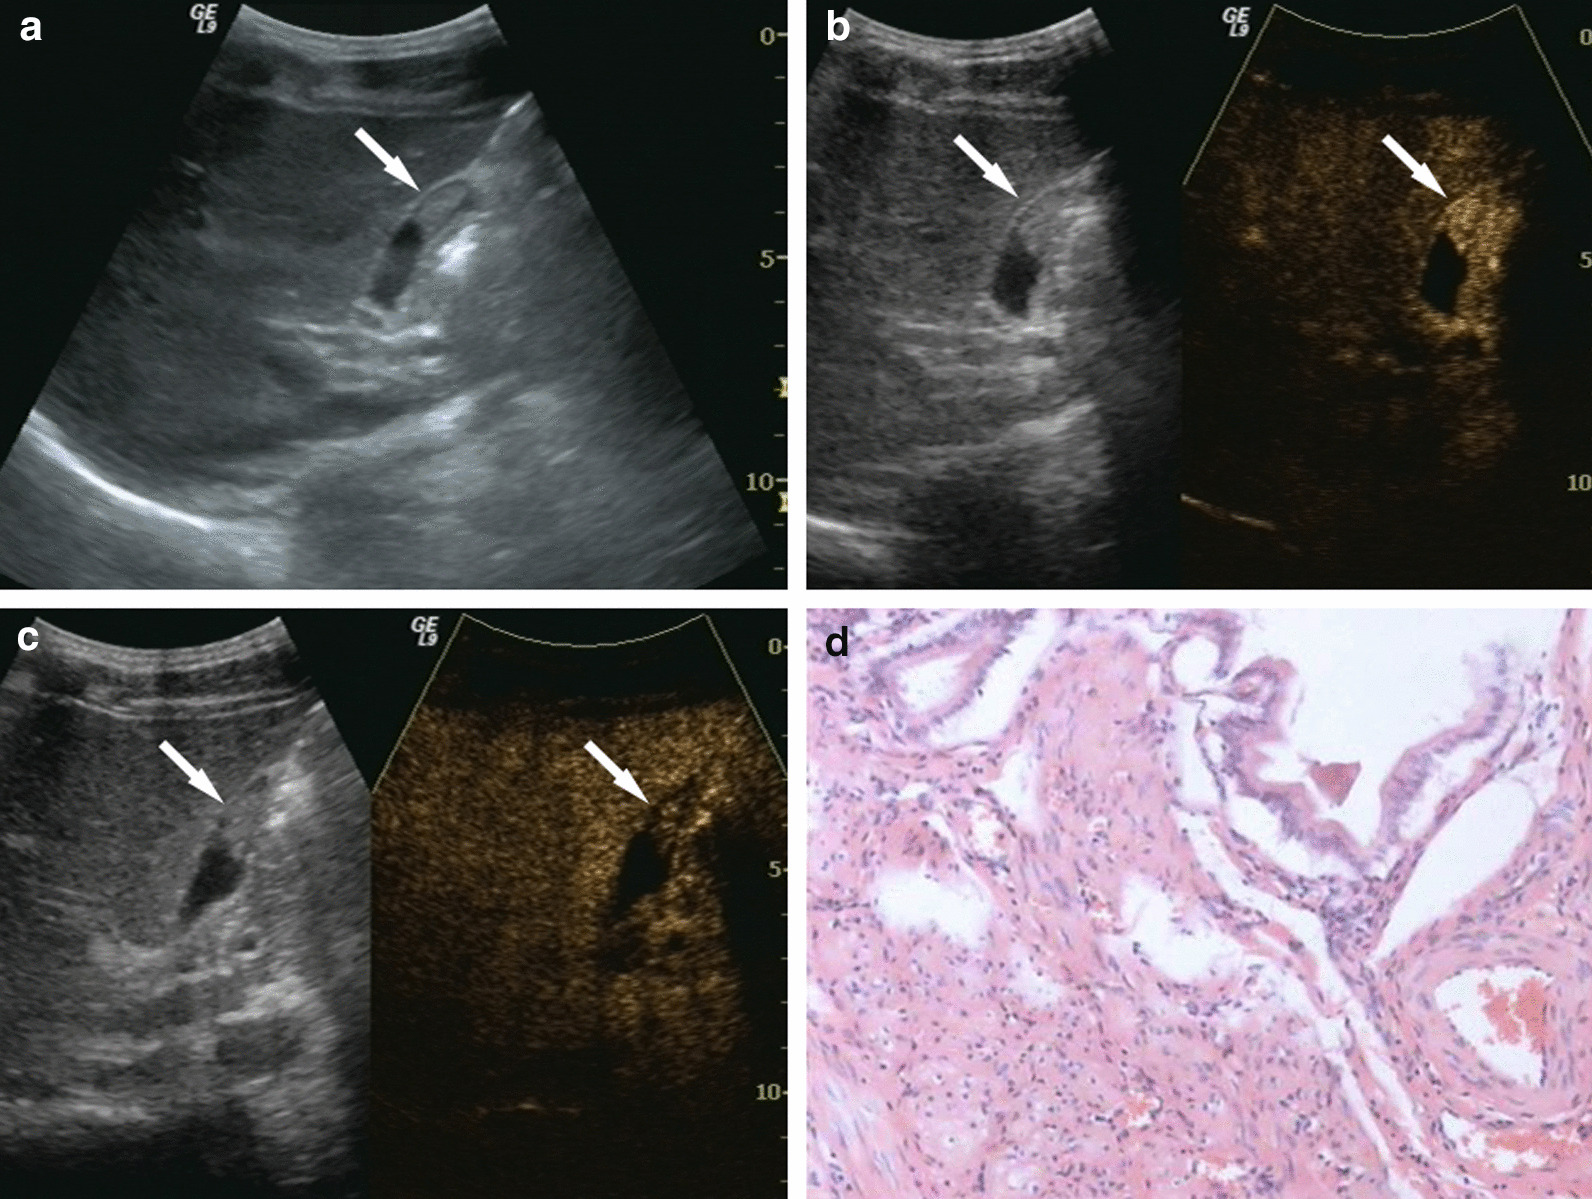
Gallbladder cholesterol polyp. a Ultrasonography (US) showed an isoechoic lesion, 1.4 × 0.6 cm in size. b On contrast-enhanced ultrasound (CEUS), the lesion showed synchronous enhancement with the gallbladder (GB) wall at 14 s in the arterial phase, and iso-enhancement in comparison to the normal GB wall. c As time passed, the contrast within the lesion showed simultaneous wash-out with the GB wall. d Postoperative pathological results: GB cholesterol polyps
